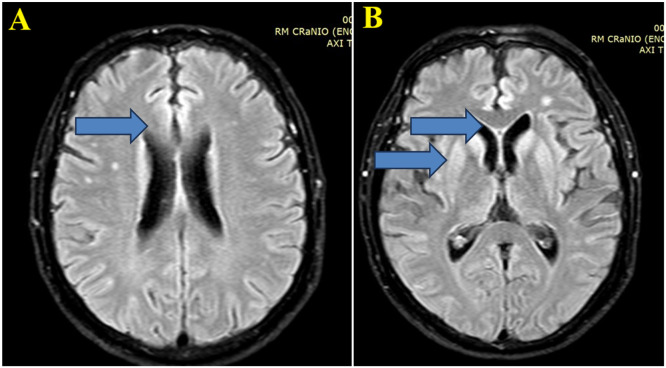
(A) Brain MRI images with Axial FLAIR sequence at the centrum semiovale show a small cortical hypersignal in the superior frontal gyrus bilaterally (blue arrow). (B) Brain MRI images at the basal ganglia level also demonstrate a symmetric and bilateral hypersignal in the caudate and putamen, as well as in the superior frontal gyri (blue arrow).
